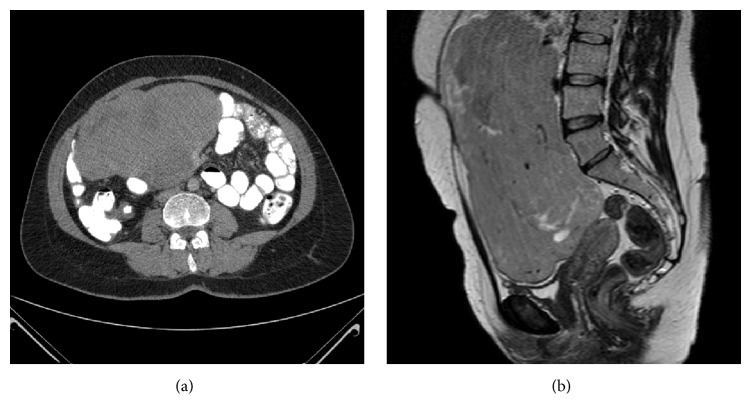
Computerized tomography (a) and magnetic resonance imaging (b) showing a solid, multiloculated, and hypervascular mass starting from the pelvis and extending to the transverse colon, 26 × 22 × 16 cm in size.
